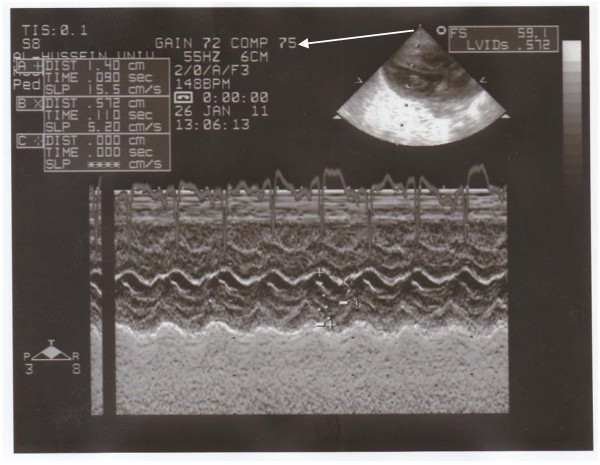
M-mode and parasternal long axis view showing interventricular septal hypertrophy in an infant of a diabetic mother.
